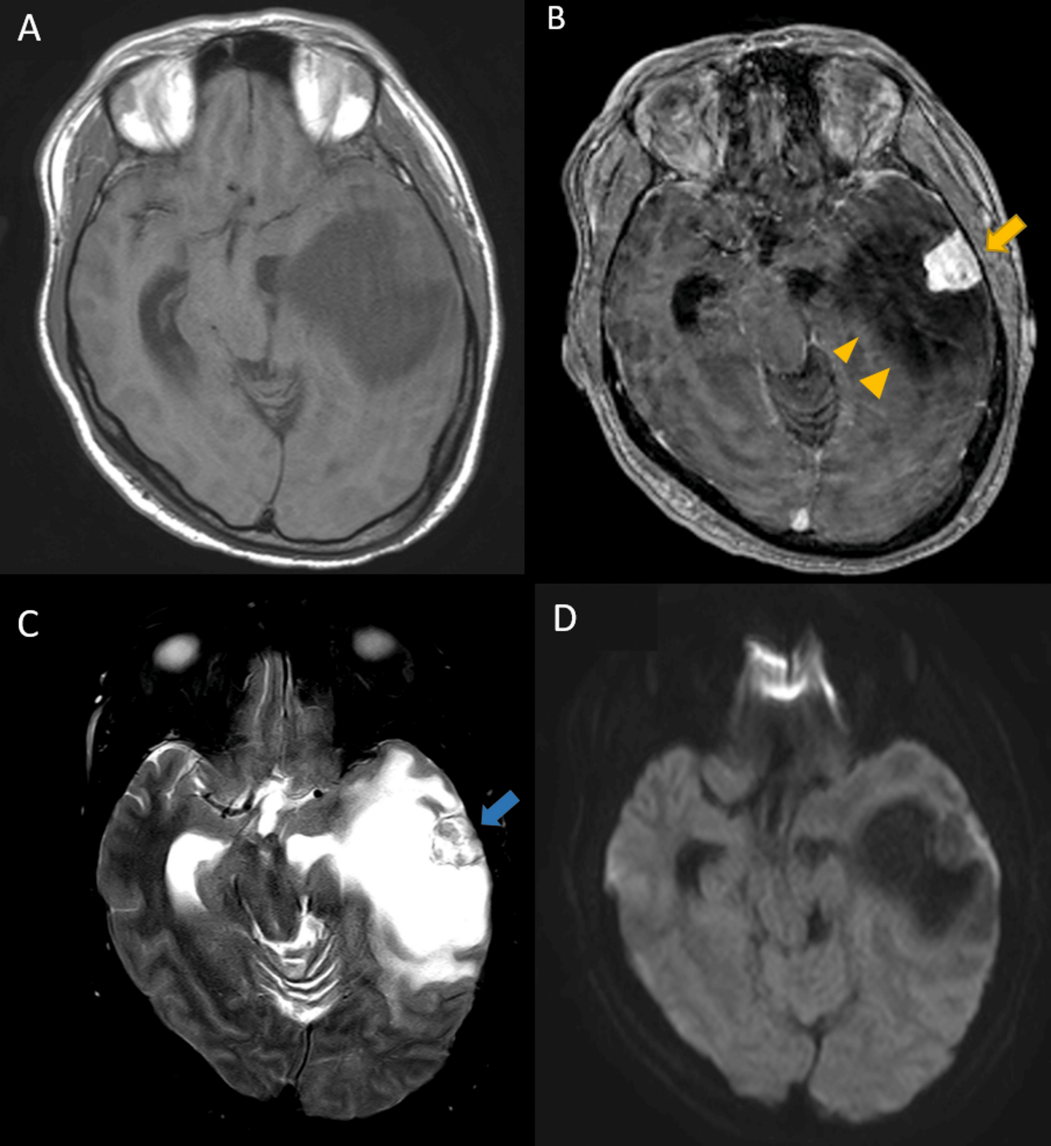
Brain MRI images of case 1 The solid part (3.1x1.9x2 cm) was isointense and the peritumoral cyst was hypointense on T1-weighted images (A). The solid part had strongly enhancing on contrast (B, yellow arrow) and heterogeneous on T2-weighted images (C, blue arrow). Peritumoral cyst (8.2x6.4x4.7 cm) was hyperintense on T2WI (C) and no contrast enhancement, including the cystic wall (B, yellow arrowhead). The tumor was showing no restriction on diffusion-weighted imaging (D). No dural tail sign was observed.
